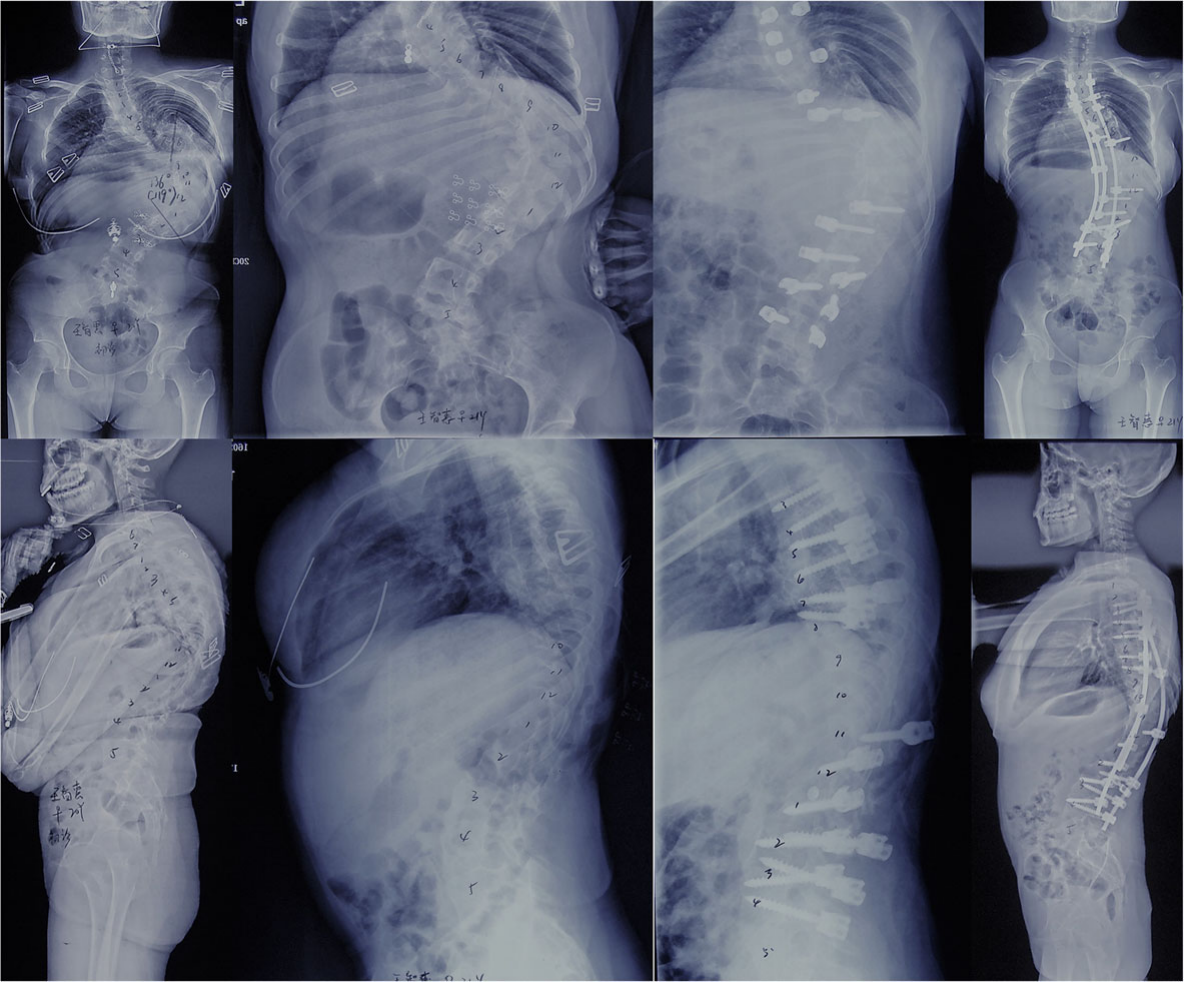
A 21-year-old female patient with congenital kyphoscoliosis had a scoliosis of 136° and a kyphosis of 85°. Major coronal curve decreased to 100° at side bending radiograph, and kyphosis to 75° at supine radiograph. After 3-week traction, scoliosis was corrected to 75°, and kyphosis to 56°. Postoperative standing radiograph demonstrated scoliosis was corrected to 60°, and kyphosis to 40°
